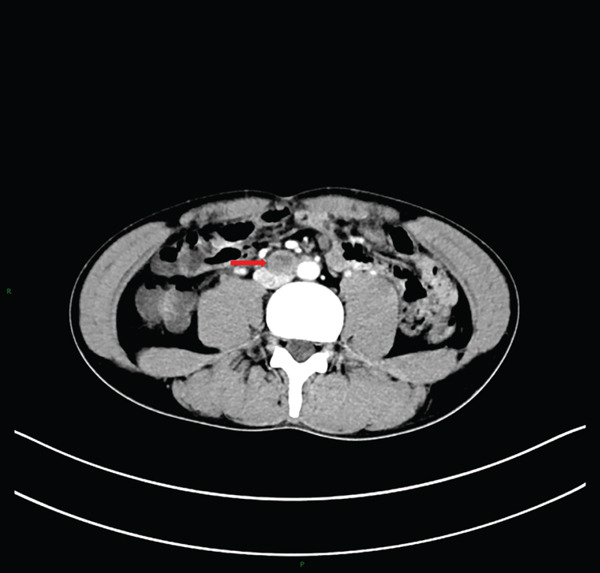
Abdominal CT image showing multiple, partially enlarged retroperitoneal lymph nodes with heterogeneous ring enhancement post‐contrast administration (arrow).
